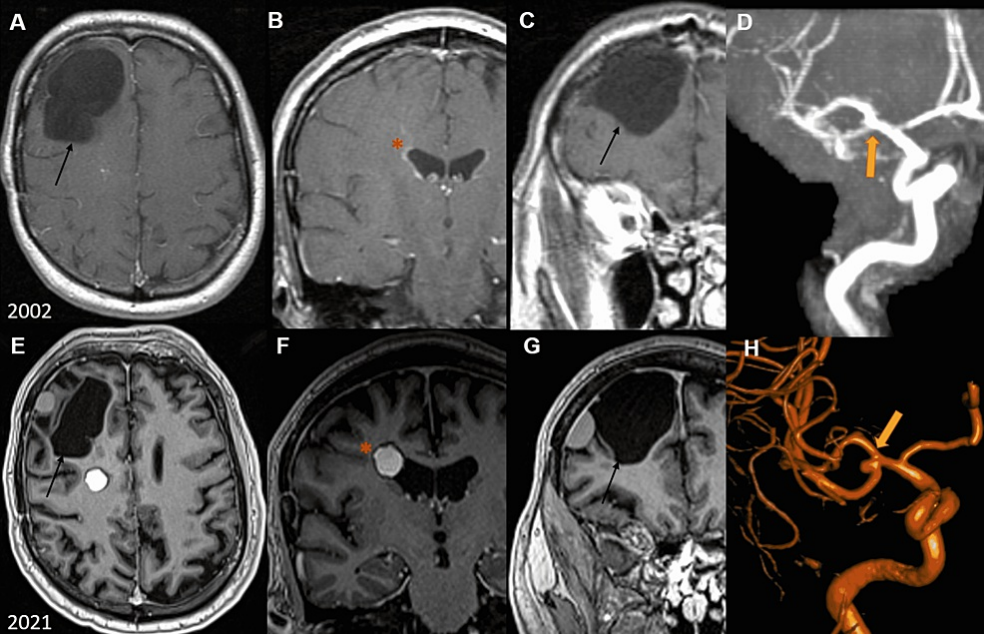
Old images vs. new images Pre-operative MRI from 2002 showing right frontal cystic lesion prior to surgical fenestration (black arrows; A, C), without evidence of cavernous malformation (red asterisk; B) and a smaller middle cerebral artery (MCA) aneurysm (yellow arrow; D). Pre-operative imaging from 2021 showing recurrence of a similarly sized right frontal cystic extra-axial non-enhancing lesion abutting the prior surgical cavity (black arrows; E, G), with a heterogeneous hyperintense mass in the right frontal corona radiata region (red asterisk; F), along with evidence of enlargement of the right MCA bifurcation aneurysm (yellow arrows; D, H).
